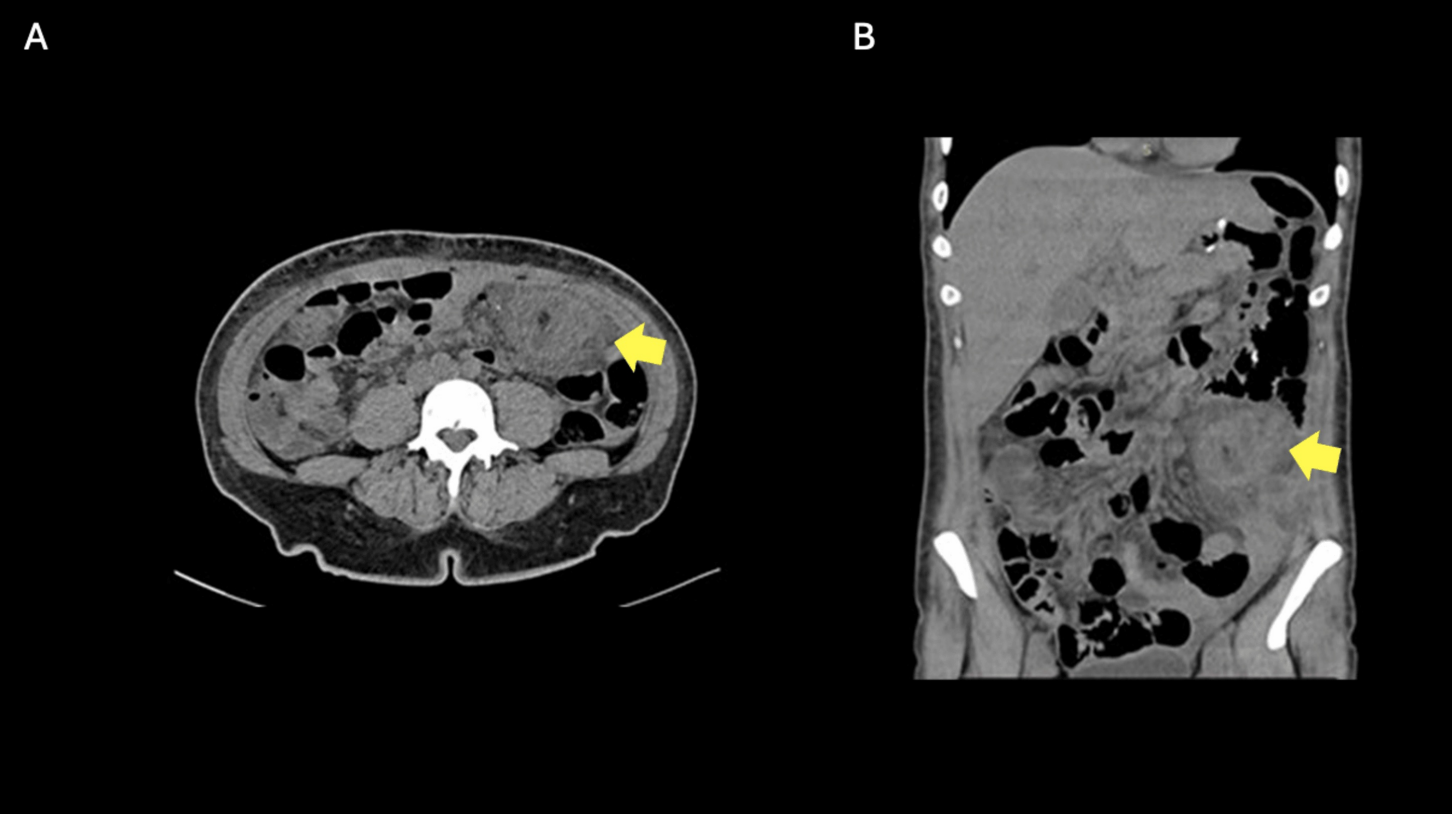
CT of the abdomen shows the circumscribed area with the inflammation centered around the omental fat at the time of diagnosis (A, axial; B, coronal view) The yellow arrow points to the area of omental infarction.
